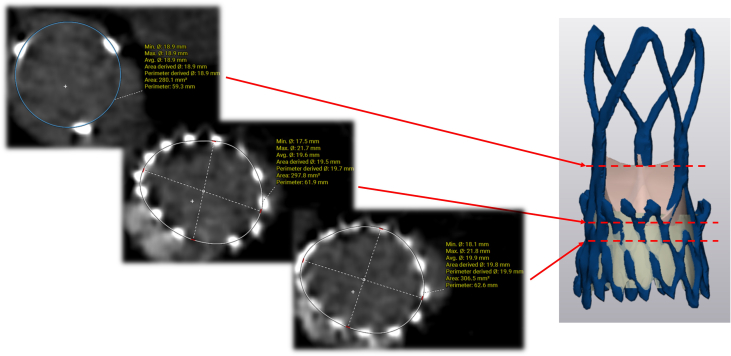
Computed Tomography Analysis Across Index Transcatheter Aortic Valve Replacement Prosthesis
